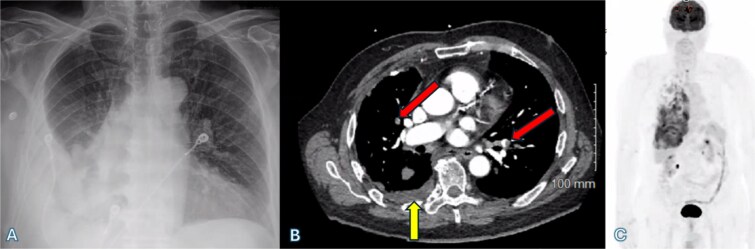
(A) Chest X-ray shows a right-sided pleural effusion. (B) Axial CT scan demonstrates pleural effusion (upward arrow) and bilateral pulmonary emboli (oblique arrows). (C) PET-CT scan shows right-sided pleural nodularity, metabolically active malignancy, and metastasis within the right pleural effusion.
